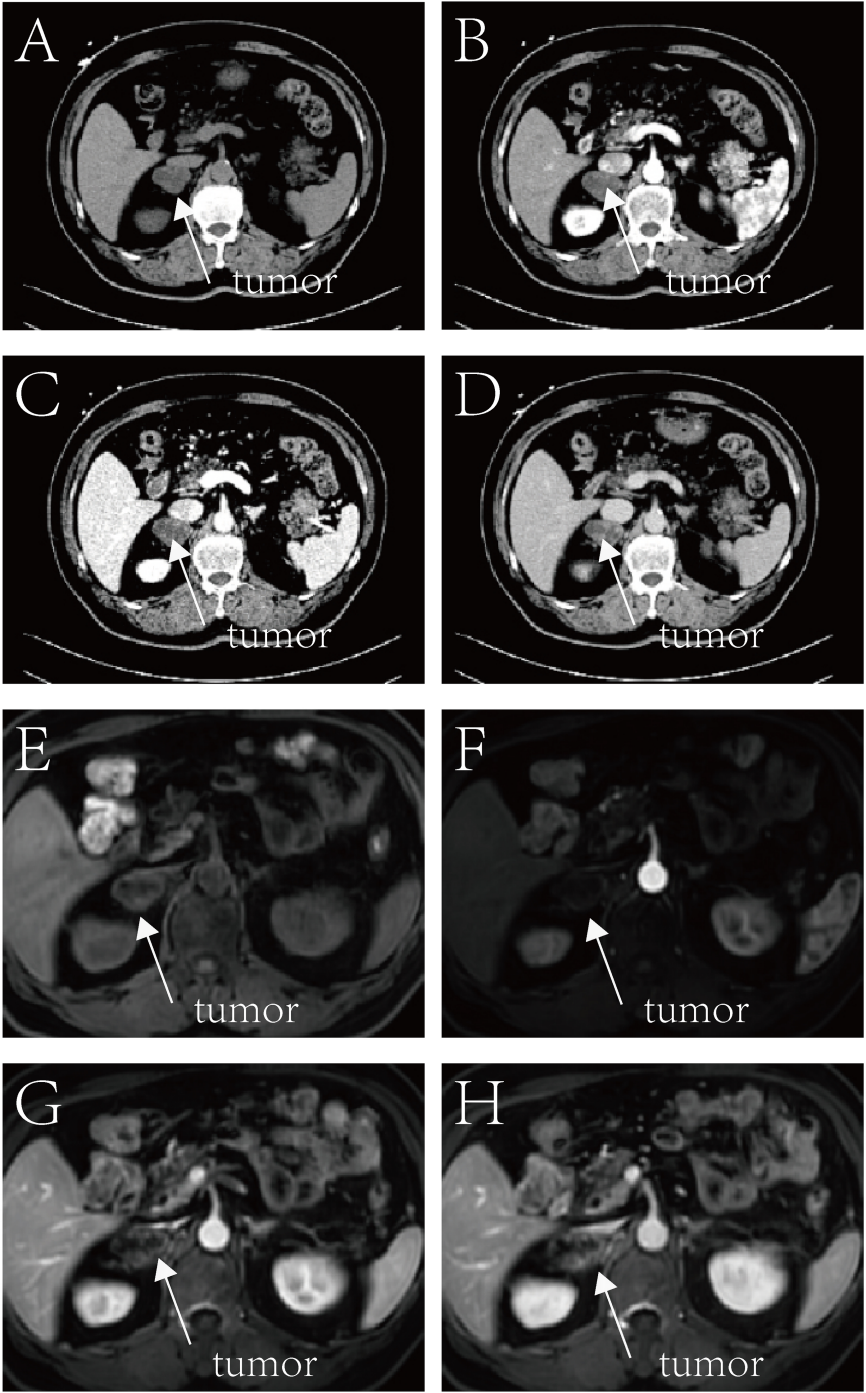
Liver plain scan enhanced hepatic arterial angiography (CTA), portal vein angiography (CTV)CT examination and plain scan enhanced MR Examination. CT (A–D) and MR (E–H) images demonstrate a mass lesion located in the right retroperitoneal region (arrows). CT imaging reveals a well-defined, heterogeneous, hypodense mass measuring approximately 2.7 × 3.7 cm, with punctate calcifications and clear demarcation from adjacent renal and adrenal structures. The lesion shows marked peripheral heterogeneous enhancement post-contrast administration. MR imaging depicts the lesion as a heterogeneous mass with mixed hyperintense signals on T1-weighted images and mixed hyper-/hypointense signals on T2-weighted images, measuring approximately 2.5 × 3.5 cm, exhibiting prominent peripheral enhancement upon contrast. Imaging characteristics suggest a neurogenic tumor, most likely schwannoma. Additionally, multiple hepatic and left renal cysts are identified, and fatty infiltration of the pancreas is evident.
